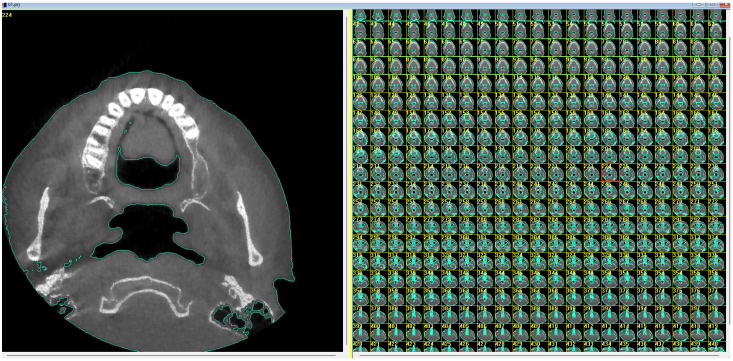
Three-dimensional soft tissue and airway modeling with CBCT and segmentation.
